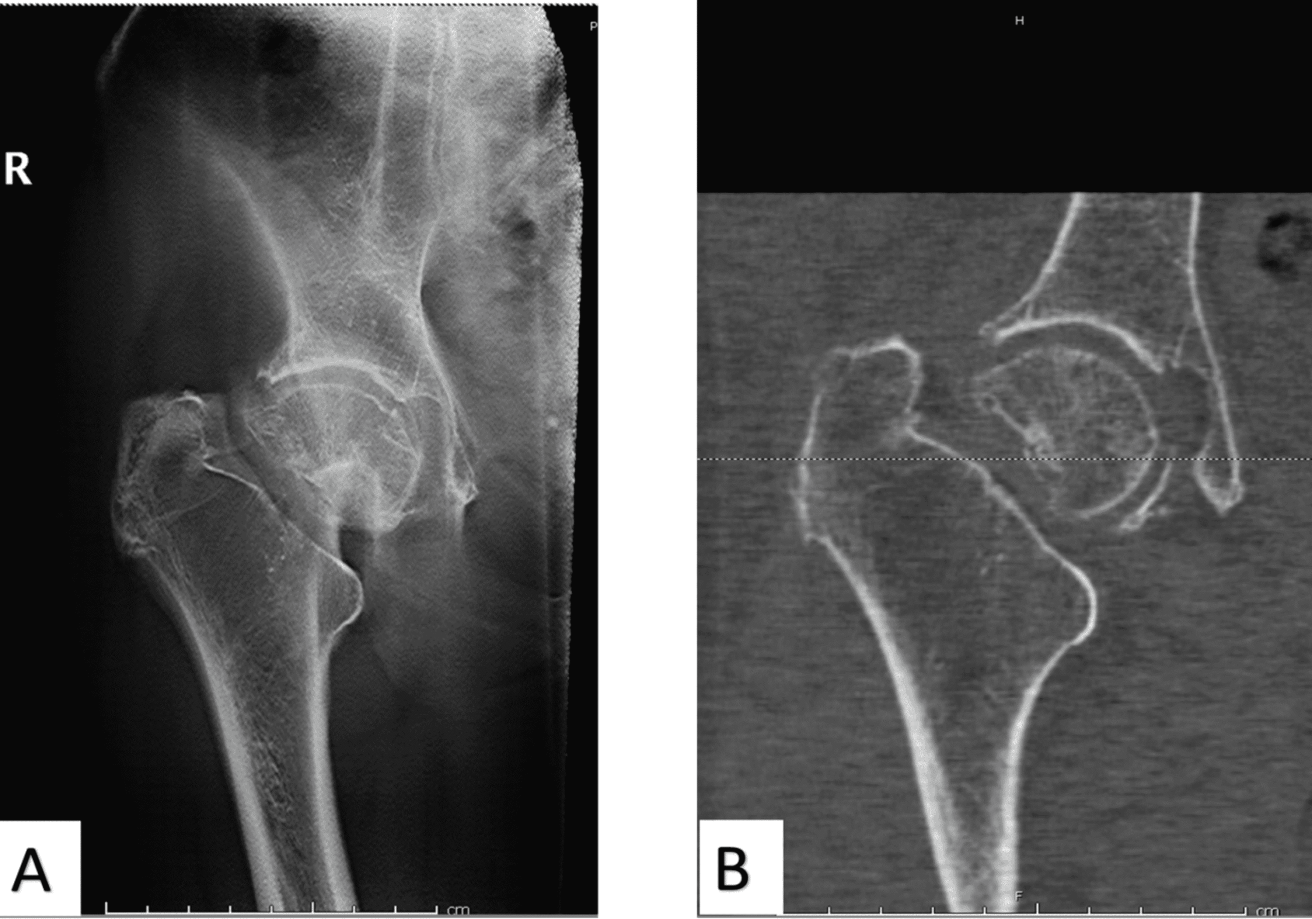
An 87-year-old female patient presented following a fall with pain and non-weight-bearing in the right lower limb. Plain radiographs showed no obvious fracture, but a tomogram was arranged, which showed a highly suspicious impacted NOF fracture (A) and was confirmed on a CT scan as the patient had contraindication for MRI (B).
